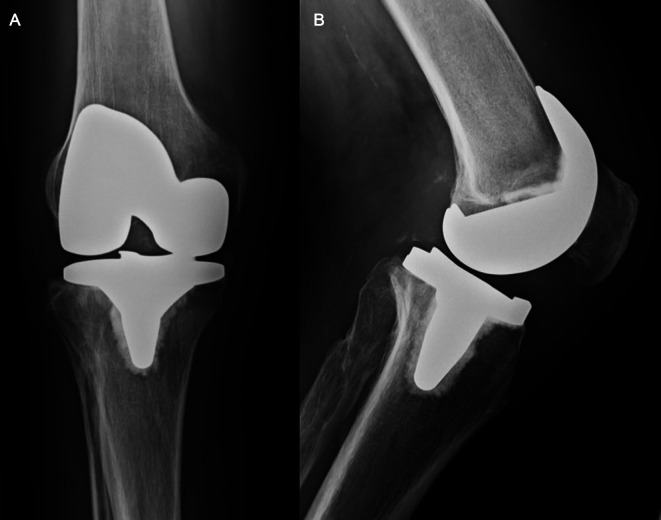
(A,B) Chronic quadriceps tendon insufficiency. Antero-posterior (A) and lateral (B) x-ray views of the knee of a patient with a chronic quadriceps tendon insufficiency. The patient sustained a traumatic quadriceps tendon rupture six weeks after primary TKA. Initial attempts of conservative treatment failed and lead to an extension lag of 20°, which was the indication for Marlex mesh reconstruction seven months after primary TKA.
